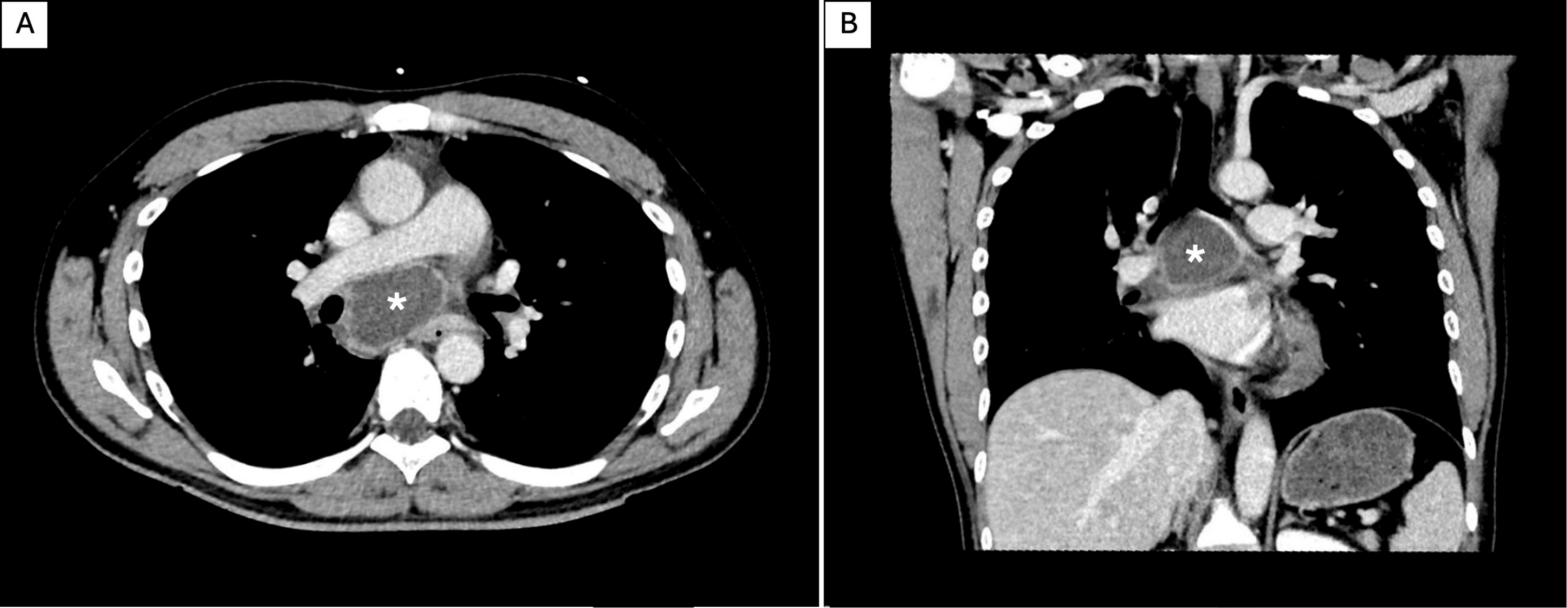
Computed tomography (CT) at presentation Contrast-enhanced CT of the chest with axial (A) and coronal (B) reformats at the level of the cyst demonstrates a rounded cystic mass measuring 5.6 cm in maximum diameter, located in the subcarinal region (white asterisk). The lesion exhibits smooth peripheral rim enhancement in venous contrast phase with a non-enhancing hypoattenuating center consistent with internal fluid content (0-20 Hounsfield Units).
